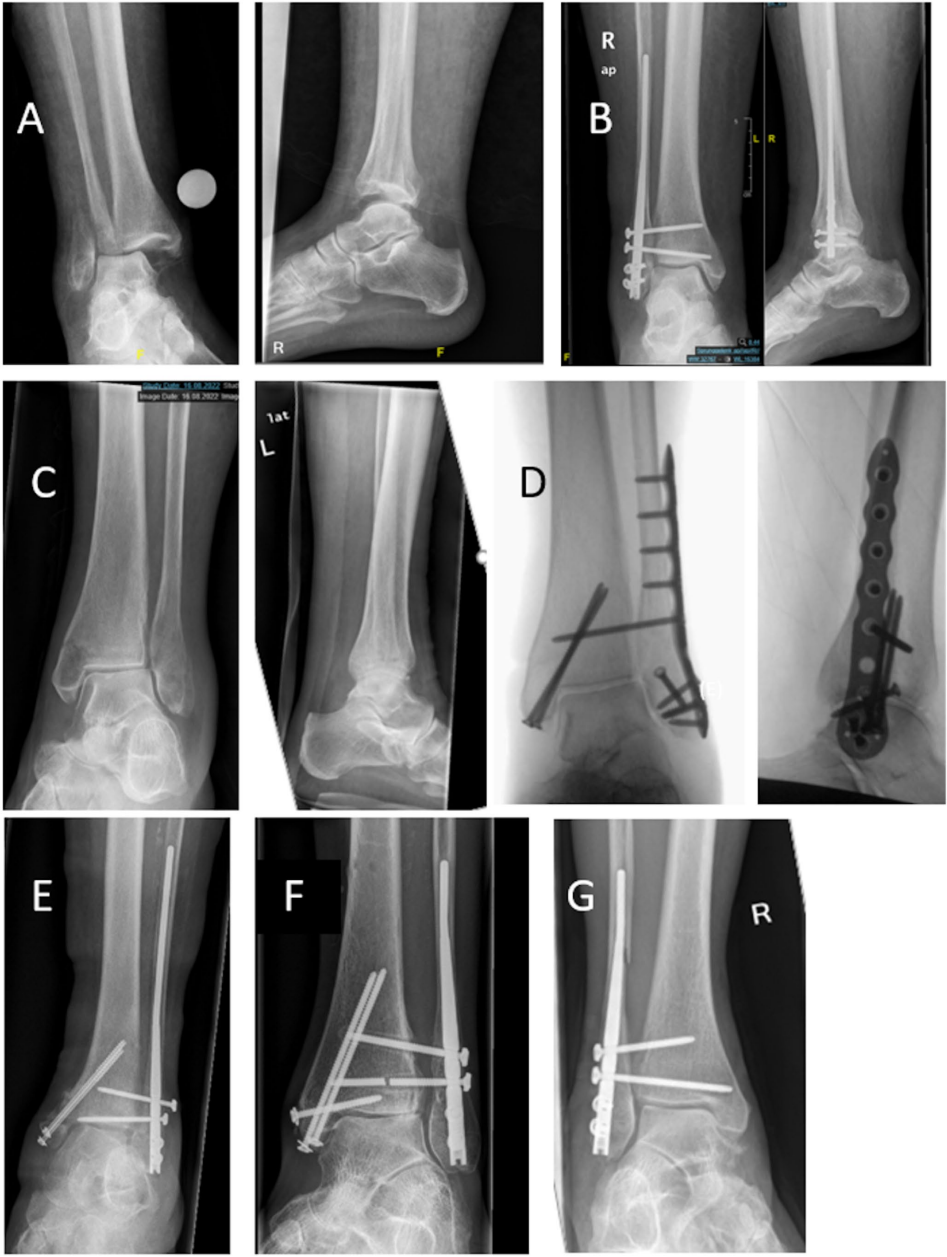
(A) Preoperative radiographs prior to fibular nail osteosynthesis., (B) Postoperative result after fibular nail fixation with interfragmentary screws. (C) Preoperative radiographs prior to subsequent plate osteosynthesis. (D) Postoperative result after open reduction and internal fixation (ORIF) with locking plate and screws. (E) Complication example after fibular nailing with screw migration/loosening. (F) Complication example with breakage of the syndesmotic screw after fibular nail fixation. (G) Complication example with diaphyseal burst (“diaphyseal blowout”) following nail insertion
